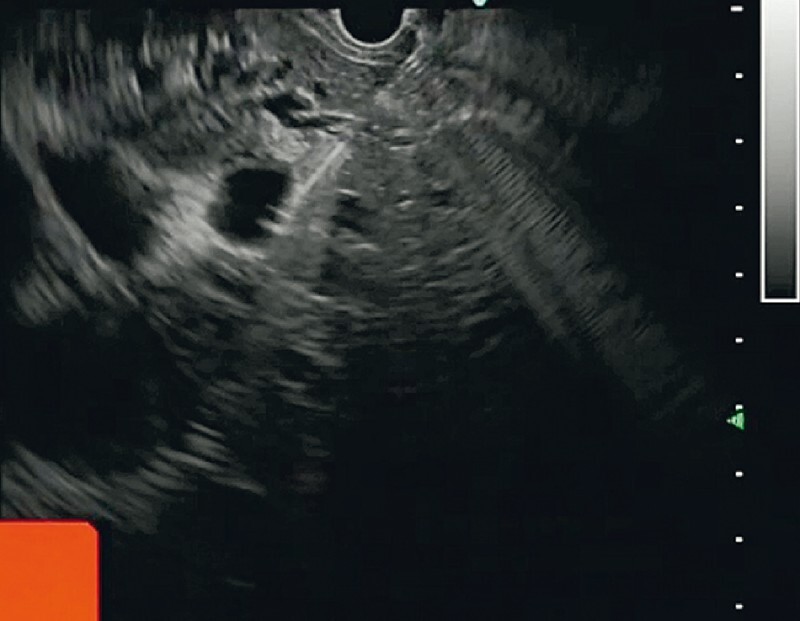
Endoscopic ultrasound (EUS) view of puncture of the dilated intrahepatic bile duct.
